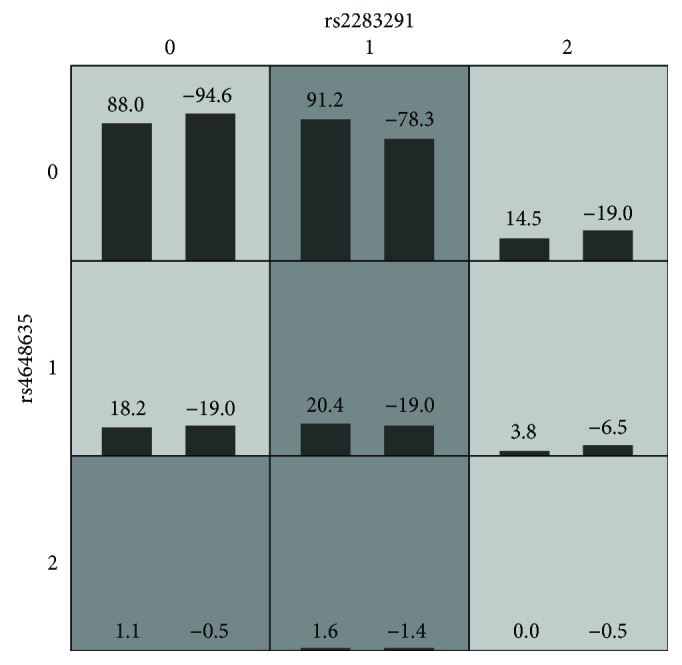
GMDR 2D interaction model in rs2283291 and rs4648635. The left bar represents the positive score and the right bar represents the negative score, and high risks are represented by dark shading and low risks by light ones. 0, 1, and 2 in the figure are the GG, GA, AA genotypes of rs2283291 and the CC, CT, TT genotypes of rs4648635, respectively.
